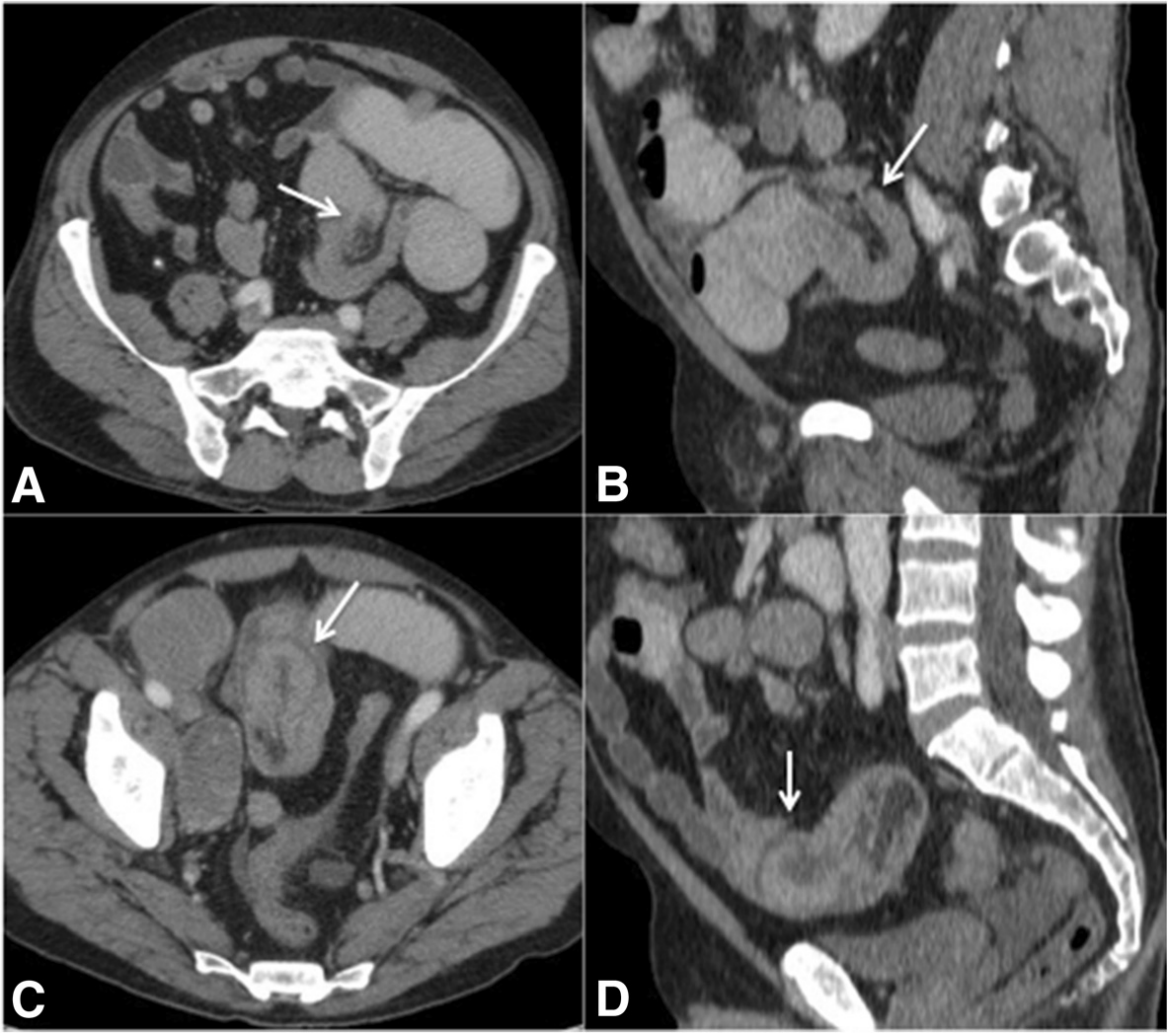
A 29-year-old man Intestinal obstruction caused by intussusception. Patient with metastatic melanoma evolving into a clinical picture of intestinal obstruction. Computed tomographic images obtained after oral and intravenous iodinated contrast show intussusception with distention of the upstream small bowel loops. (A) Axial and (B) sagittal images showing the proximal portion of intussusception and invagination of the mesenteric adipose tissue toward its interior (arrows). (C) Axial and (D) sagittal images showing the distal portion of intussusception with parietal thickening of the invaginated small intestinal loops (arrows), which was confirmed as a melanoma metastasis after surgical resection.
